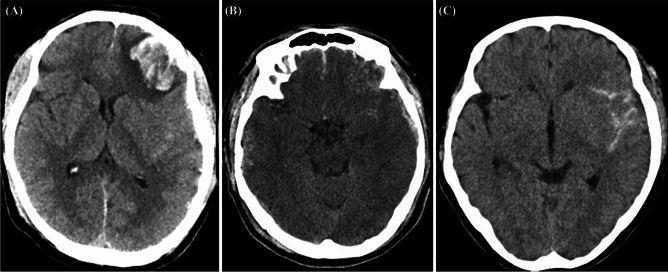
Intracerebral hemorrhage types on CT. (A) Intraparenchymal hemorrhage, indicated by uniformly dense lesions within brain tissue. (B) Petechial hemorrhage, with characteristic ‘salt and pepper’ patterning alongside subarachnoid hemorrhage. (C) Subarachnoid hemorrhage, shown by dense lesions in subarachnoid and sulcal spaces.
